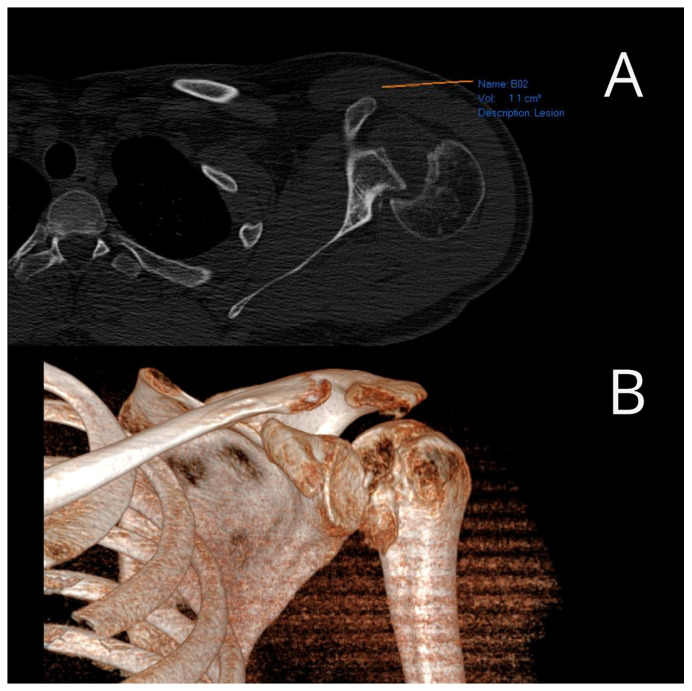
Preoperative axial (A) and three-dimensional (B) CT image of a reverse Hill–Sachs lesion (photo from the personal archive of intraoperative photos of the author).
